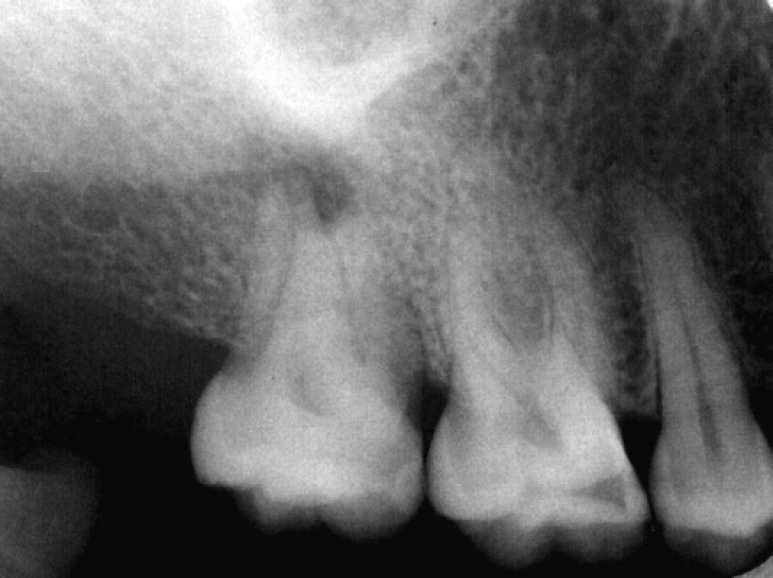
Preoperative radiograph demonstrated periapical radiolucency and abnormal roots
